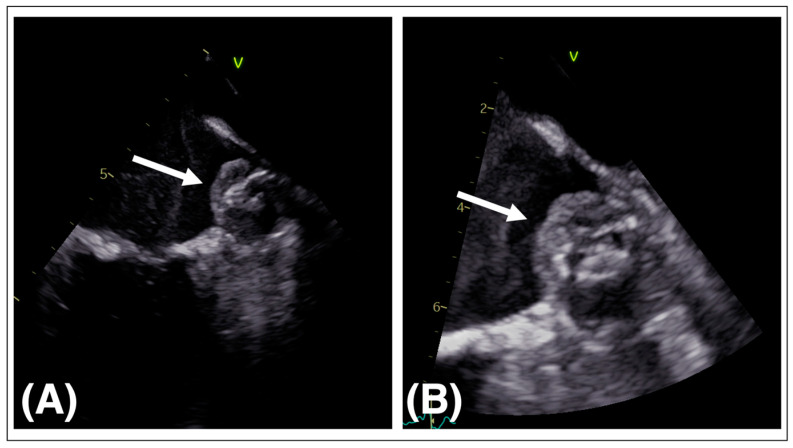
Device related thrombosis. (A,B) 2D TEE of the LA after LAAO and visualization of device related thrombosis on the atrial surface of the WATCHMAN™ device (white arrows). TEE transesophageal echocardiography, LA left atrium, LAAO left atrial appendage occlusion.
